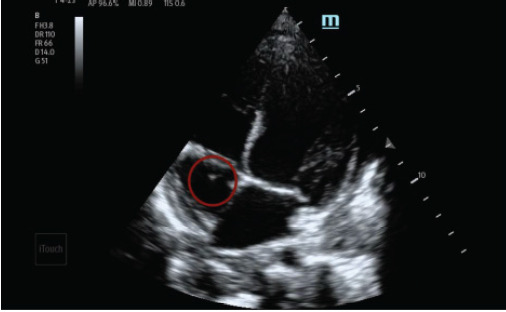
Hyperechoic structure (circled) seen in right atria during systole attached to tricuspid valve in apical four view of a point-of-care ultrasound.
